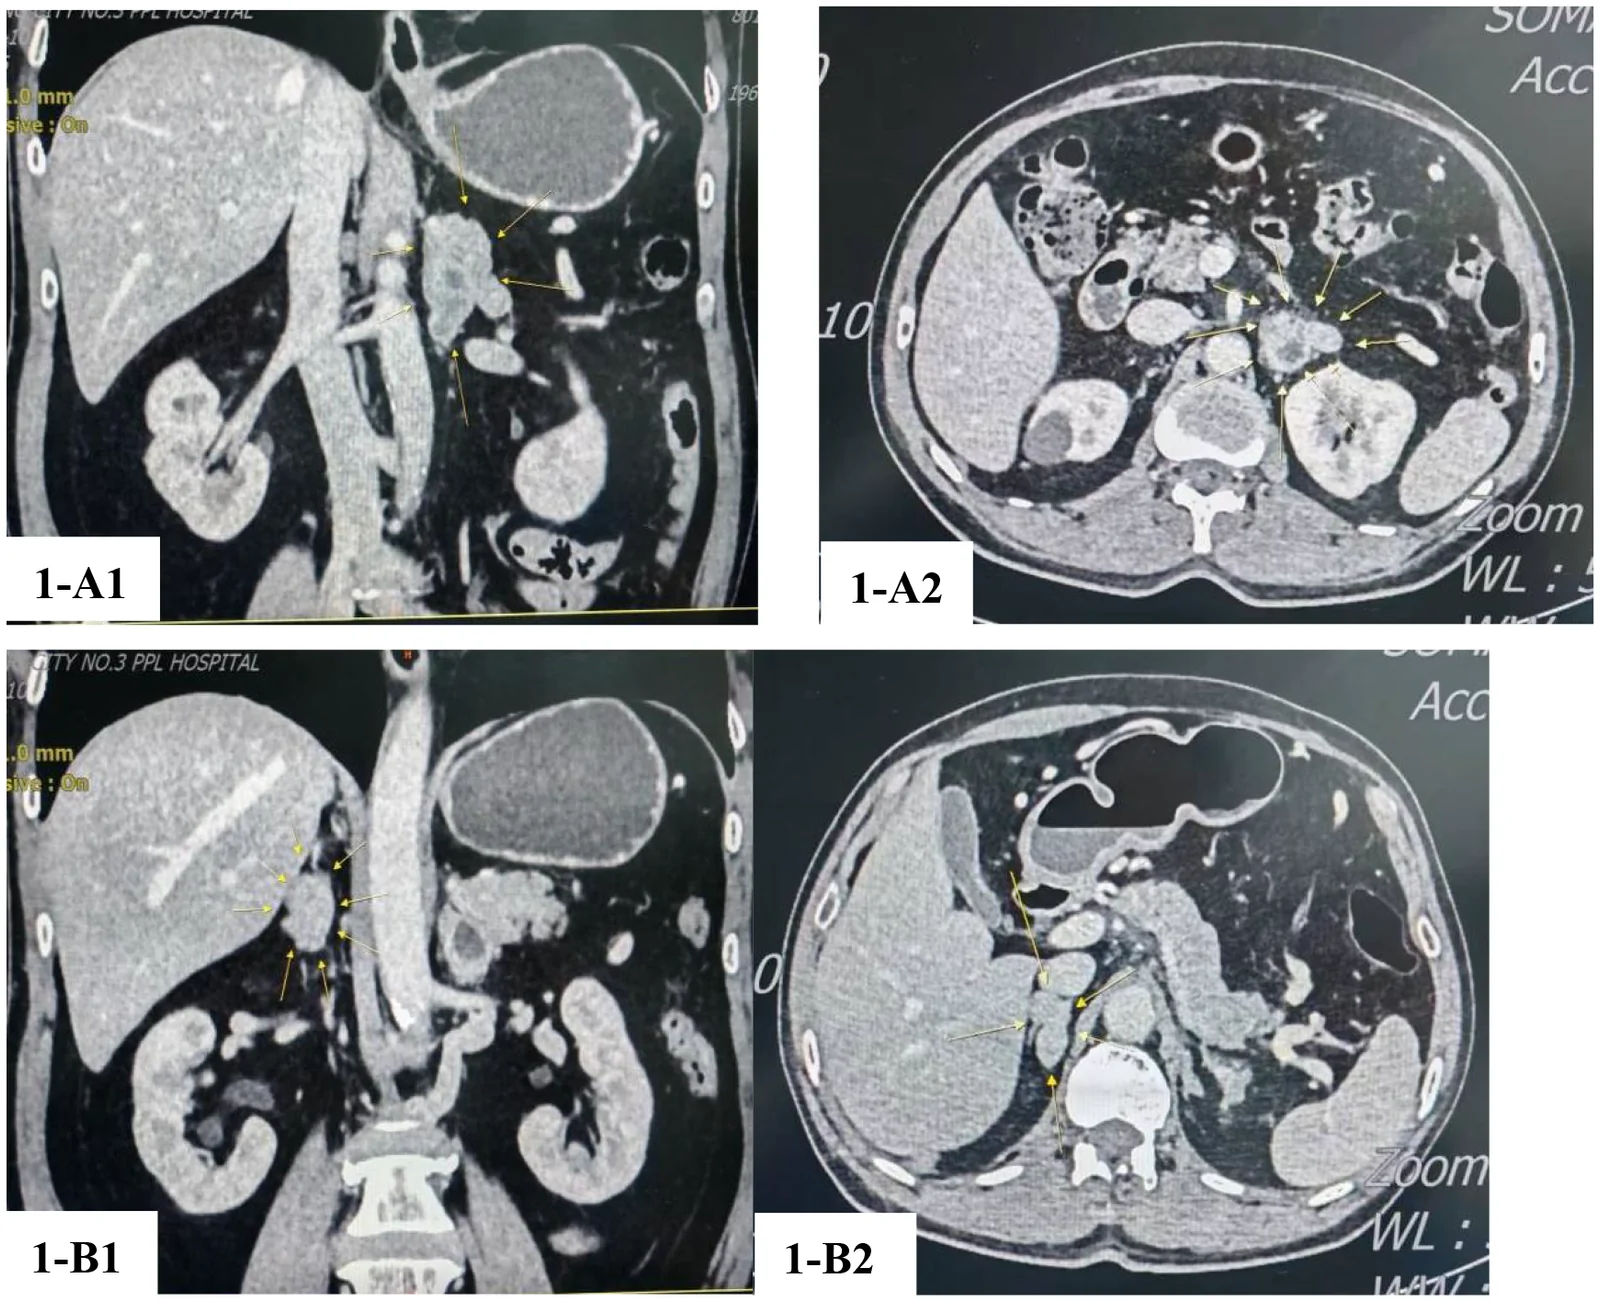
Contrast-enhanced abdominopelvic CT images. [(A1), coronal plane; (A2), axial plane] A well-circumscribed slightly hypoattenuating nodule is identified inferior to the left adrenal gland and medial to the mid-left kidney, demonstrating prominent nodular and peripheral ring enhancement (yellow arrow). [(B1), coronal plane; (B2), axial plane] A separate slightly hypoattenuating nodule is visualized within the right adrenal fossa (yellow arrow).*CT* computed tomography.
